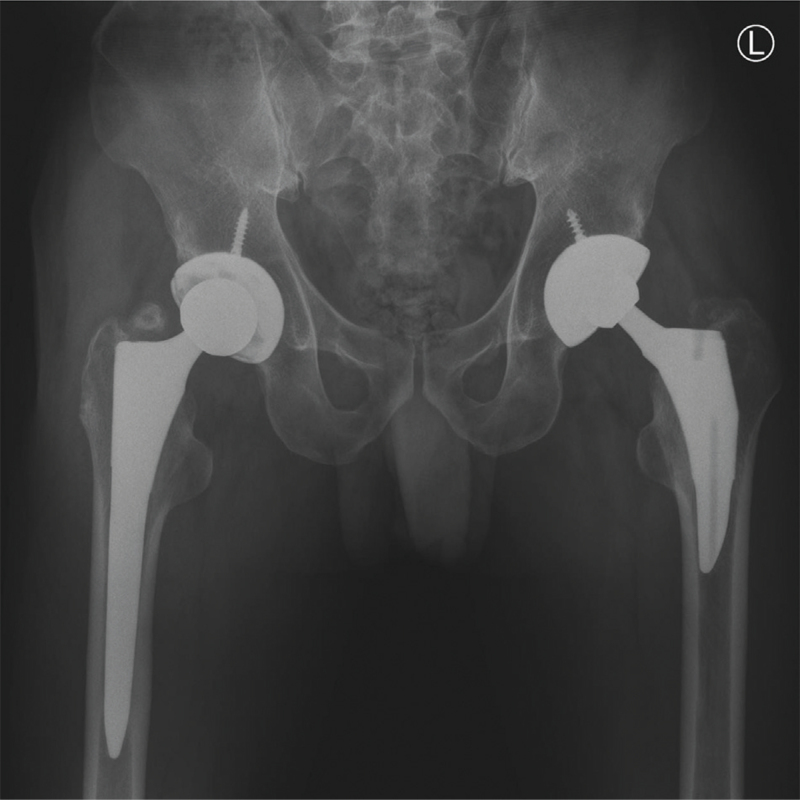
AP radiographs of the pelvis taken 2 years post-surgery following revision of hip components. AP = anteroposterior.
